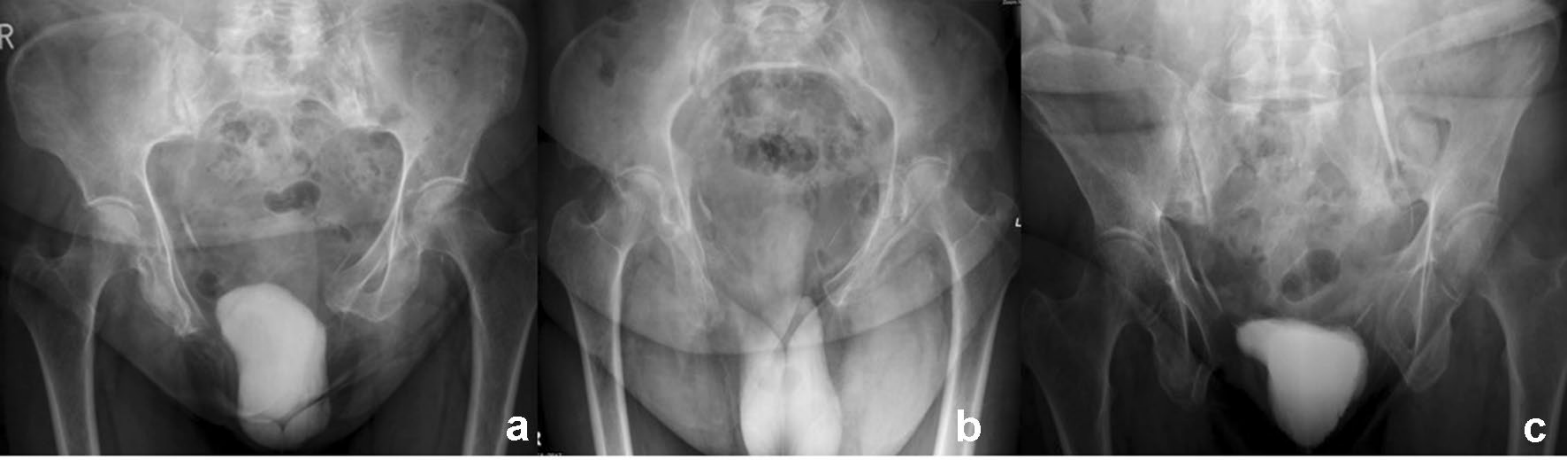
Pre-operative conventional X-rays with simultaneous cystogram. From left to right: AP view (a), inlet view (b), outlet view (c). The malunion of the pubic rami is best seen in the AP view, (a) the bladder herniation in the inlet view (b)
